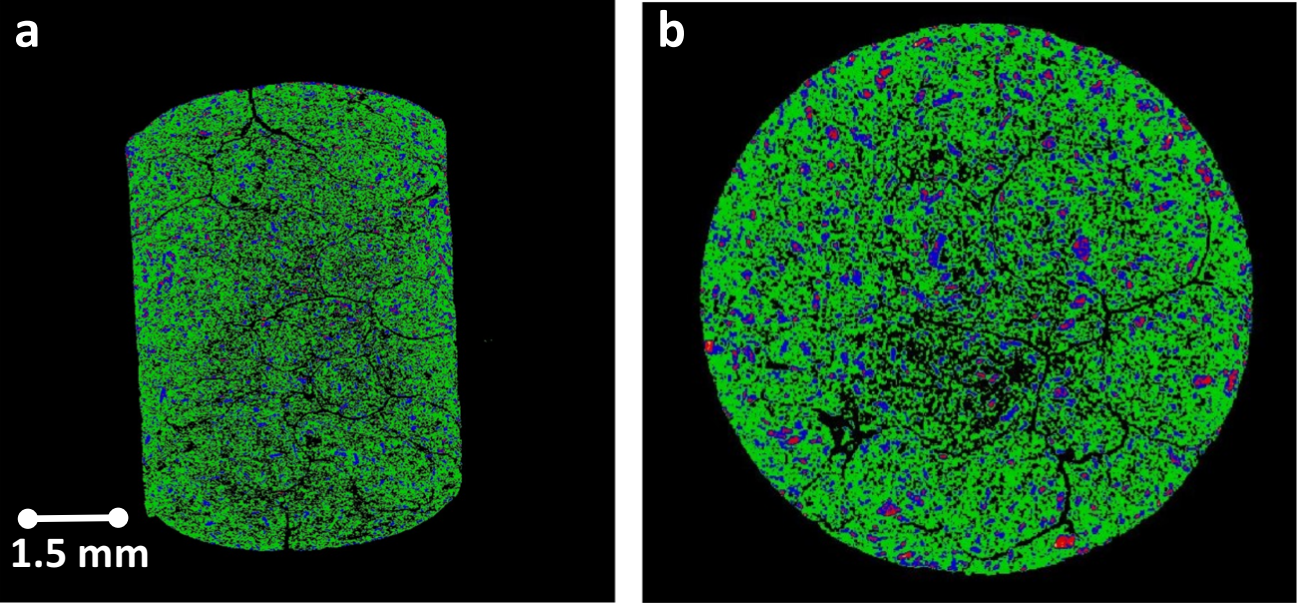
X-ray micro-tomography of the sagittal and diametric images of compacted F-5 Liqui-Tablet formulation a side of the tablet b top of the tablet. Note: This tomography technique is based on the differential absorbance of X-rays between materials of differing electron density. The color code is therefore from the density histogram. The green coloration represents the bulk of the material from the formulation which for F5 is the carrier composition of 50% Avicel PH101 & 50% Neusilin US2. The pink coloration represents the naproxen content with the blue color surrounding it representing the liquid vehicle. The black coloration within the Liqui-Tablet representing the pore spaces as well the demarcations of the individual pellets compressed together to form the Liqui-Tablet
